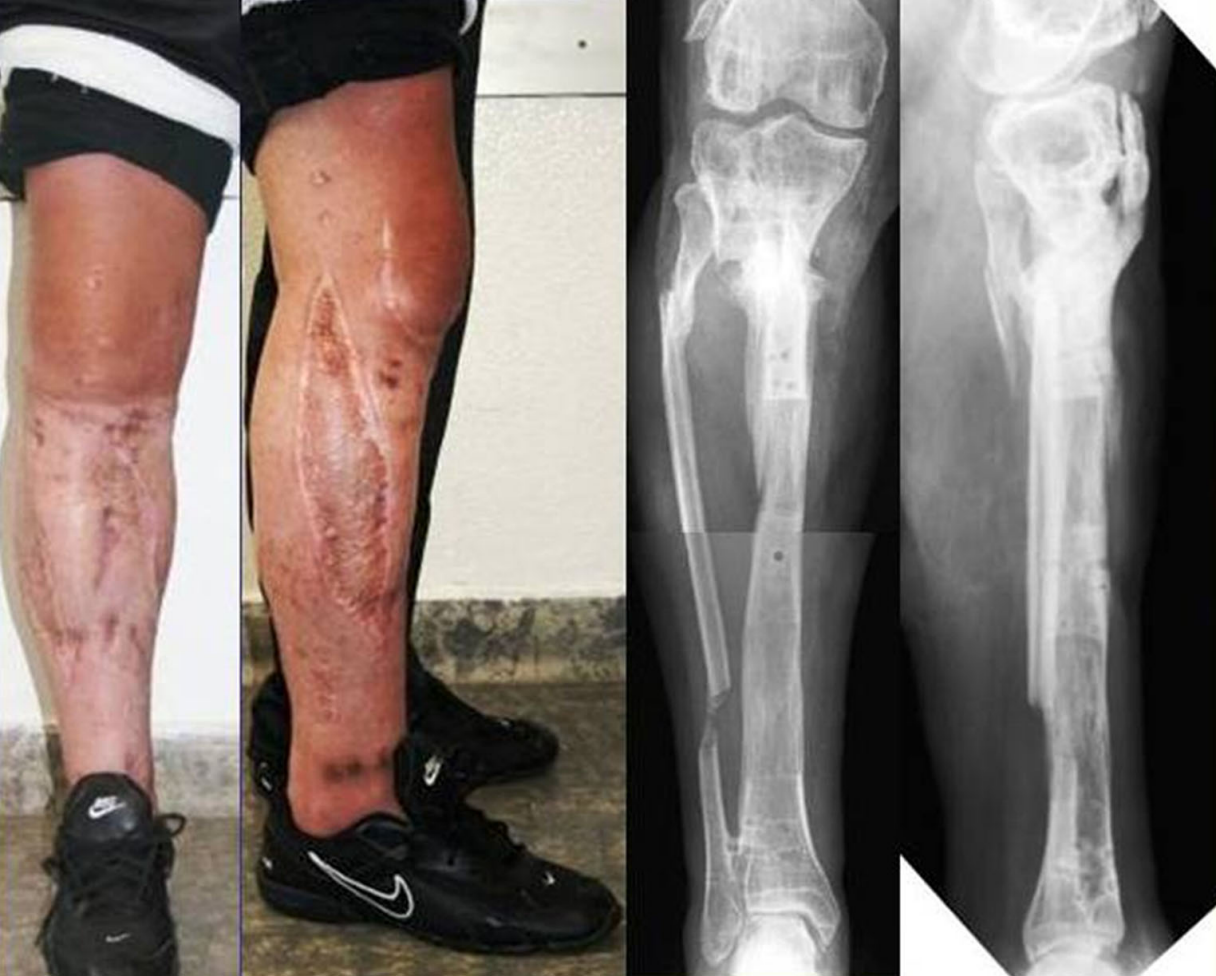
Clinical and radiographic follow-up images obtained 3 years after tibial frame removal. Treatment time, 16 months; lengthening amount, 140 mm; lengthening index (months/cm), 1.14. Mechanical axis deviation was 8 mm medial to the center of the knee joint line. Patient resumed full weight bearing without support and with no discomfort
